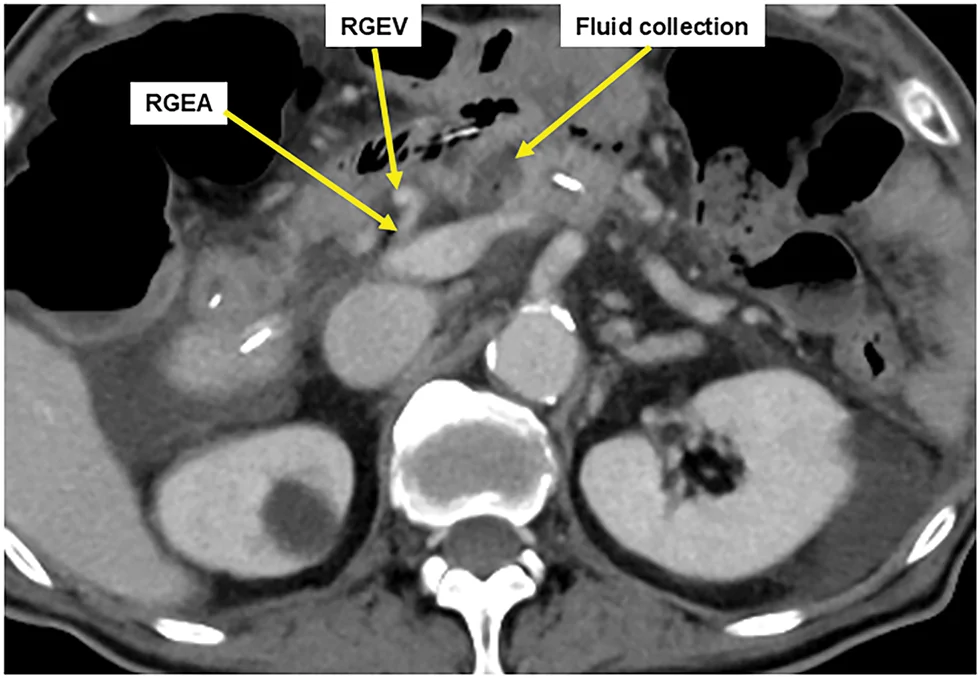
Postoperative CT findings. CT demonstrated a localized fluid collection on the cranial side of the pancreatojejunostomy, whereas only a minimal amount of fluid was observed around the preserved GDA–RGEA axis and RGEV, with no evidence of pseudoaneurysm or vascular compromise. GDA, gastroduodenal artery; RGEA, right gastroepiploic artery; RGEV, right gastroepiploic vein
